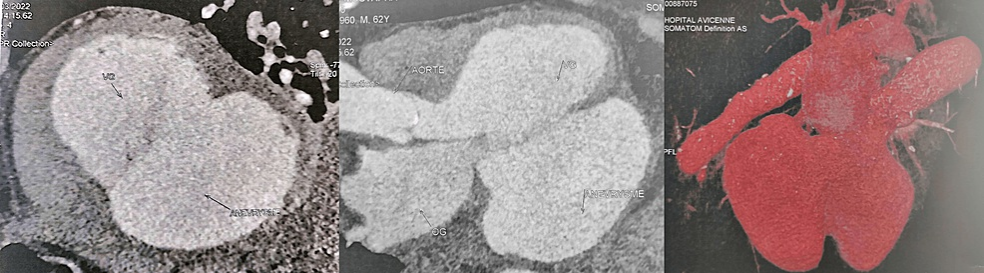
Cardiac CT showing a giant thin-walled (3 mm) submitral aneurysm (97 x 74 mm) free of thrombus with a wide neck (67 mm) and no thrombus or pericardial effusion.. CT: computed tomography.
